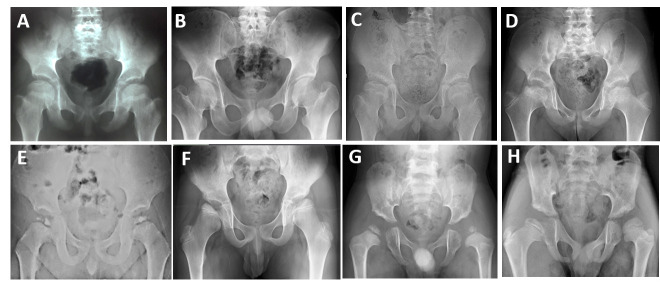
Radiographs of patients with biallelic variants in *COL9A1* (P7, P9) and *COL9A3* (P10) with Stickler types IV and VI are shown in the first line. P7 at 9 years (A) and P9 at 12 years (C) display bilateral, slightly irregular acetabula, flattened and slightly irregular proximal femoral epiphyses, and broad, shortened femoral necks with coxa valga. P7 at age 18 (B) shows a narrowed joint space, a slightly small femoral head and a shortened femoral neck with a high greater trochanter. In P10, at age 9 (D), an irregular acetabulum and a hypoplastic and irregular femoral head epiphysis, more pronounced on the right side and coxa vara, are noted. In the second line, pelvic radiographs of P11 and P12, who have a monoallelic variant in *COL11A1* are shown. Note the fragmentation of the proximal femoral epiphysis in 7-year-old P11 (E), and hypoplasia of the medial part of the proximal femoral epiphysis in 9-year-old P12 (F). P13, who has a biallelic variant in *COL11A2*, is shown in pelvic radiographs at ages 3 and 8. Note the oblique acetabulum and small, hypoplastic femoral head (G), as well as the coxa vara and straight femoral head (H).
